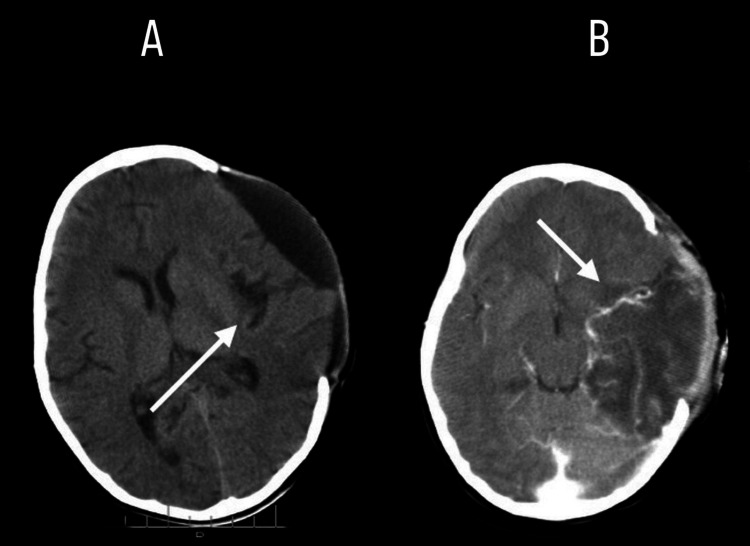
(Postoperative CT) 6A: Displayed diffuse hyperintensity on T2/FLAIR in left temporal lobe (indicated by the white arrow). 6B: Improvement of edema, and stabilized midline structures post-DC (indicated by the white arrow).
